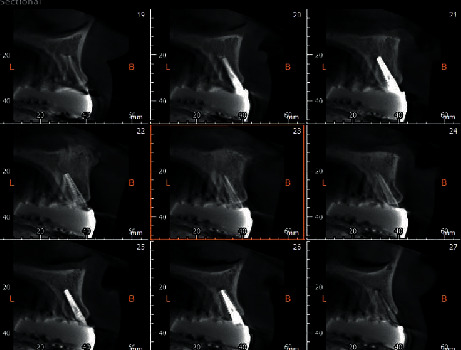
A CBCT image of one of the included cases.
